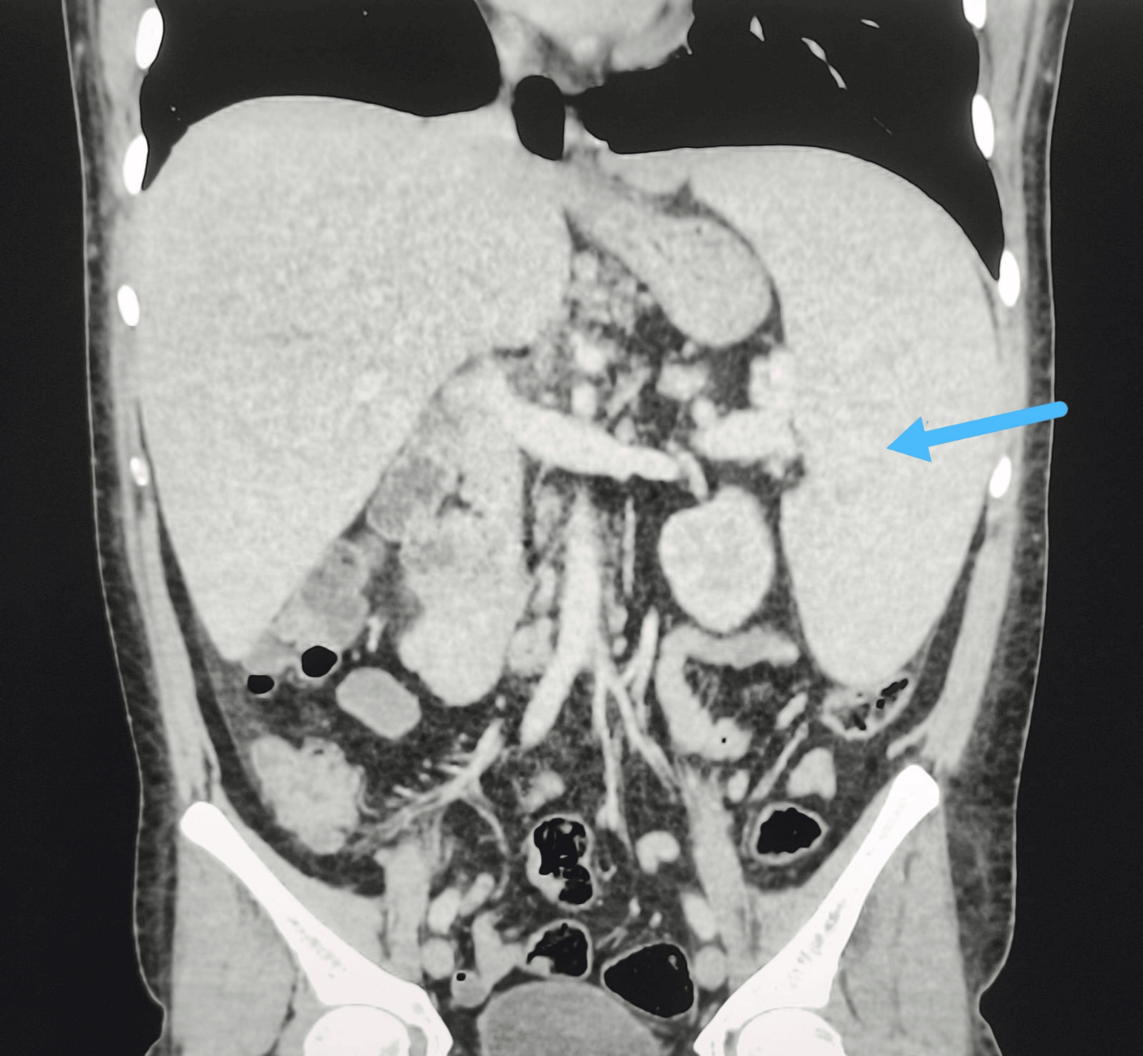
Abdominal CT scan demonstrating homogeneous splenomegaly The blue arrow indicates the enlarged spleen of the patient.
